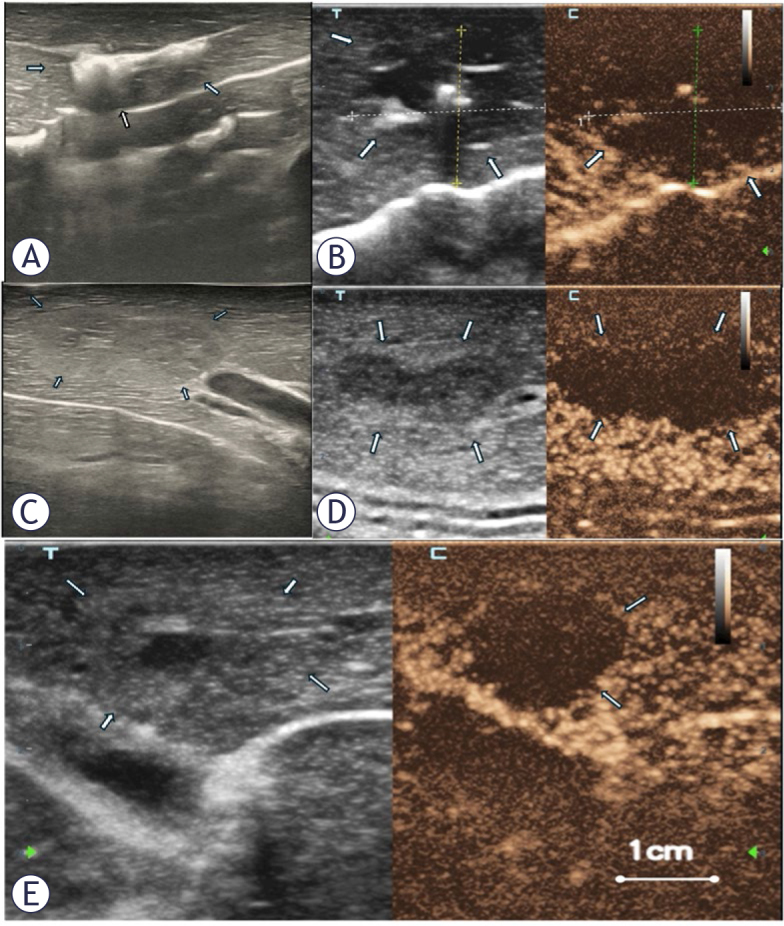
Ultrasound and CEUS findings after irreversible electroporation ablation. A scan obtained during ablation (A) confirms that the position of the electrode is appropriate. Scan obtained immediately after irreversible electroporation application (B) showing a hypoechoic ablative zone with well-demarcated margins surrounding the electrode. It appears as an avascular area in the arterial phase immediately after ablation. Scan obtained 48 h after irreversible electroporation (C) showing that the echogenicity of the central ablation zone increases, whereas the surrounding area is a ring-shaped hyperechoic band. Long axis view (D) and short axis view (E) showing that the ablation zone appears as a hypo-enhanced area in the arterial phase, and there is internal enhancement within the ablation zone 48 h after irreversible electroporation ablation.
